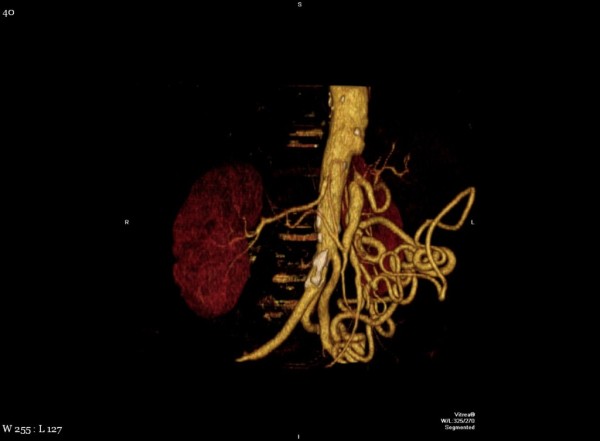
CT reconstruction showing vascular anomaly.
